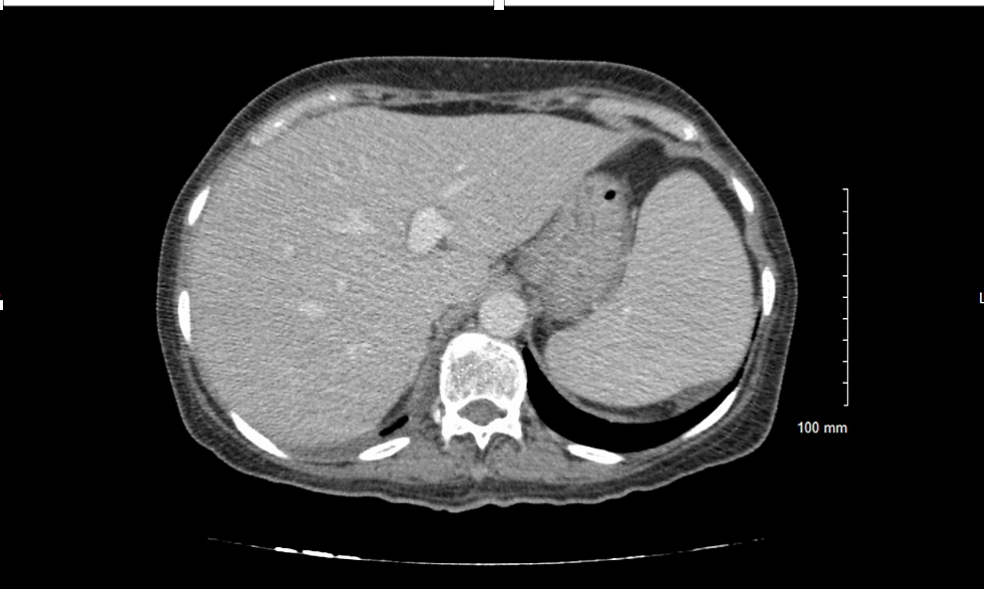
CT scan of the abdomen showing hepatosplenomegaly
